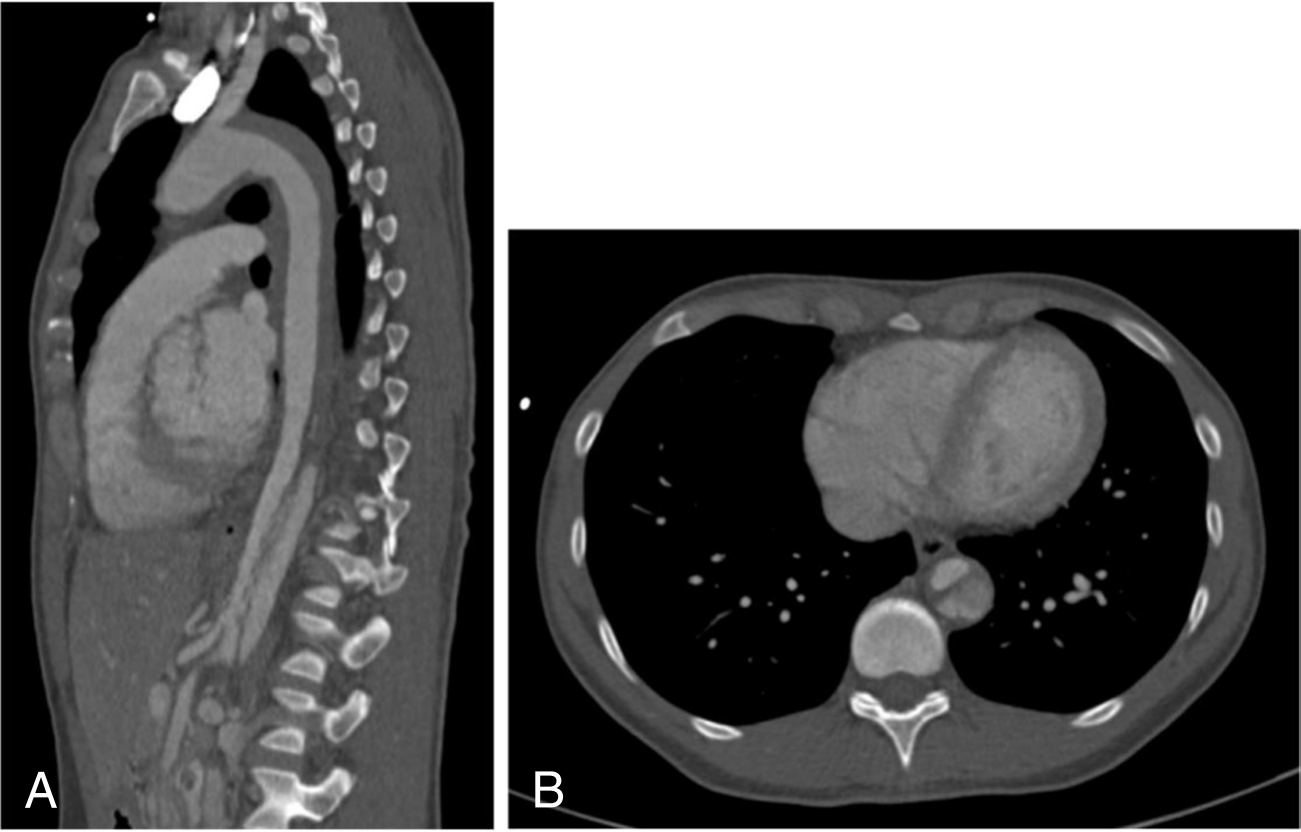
Initial CTA (A sagittal, B axial) showing an intramural hematoma followed by a true aortic dissection reaching from the left subclavian artery to the aortic bifurcation. CTA, computed tomography angiography
